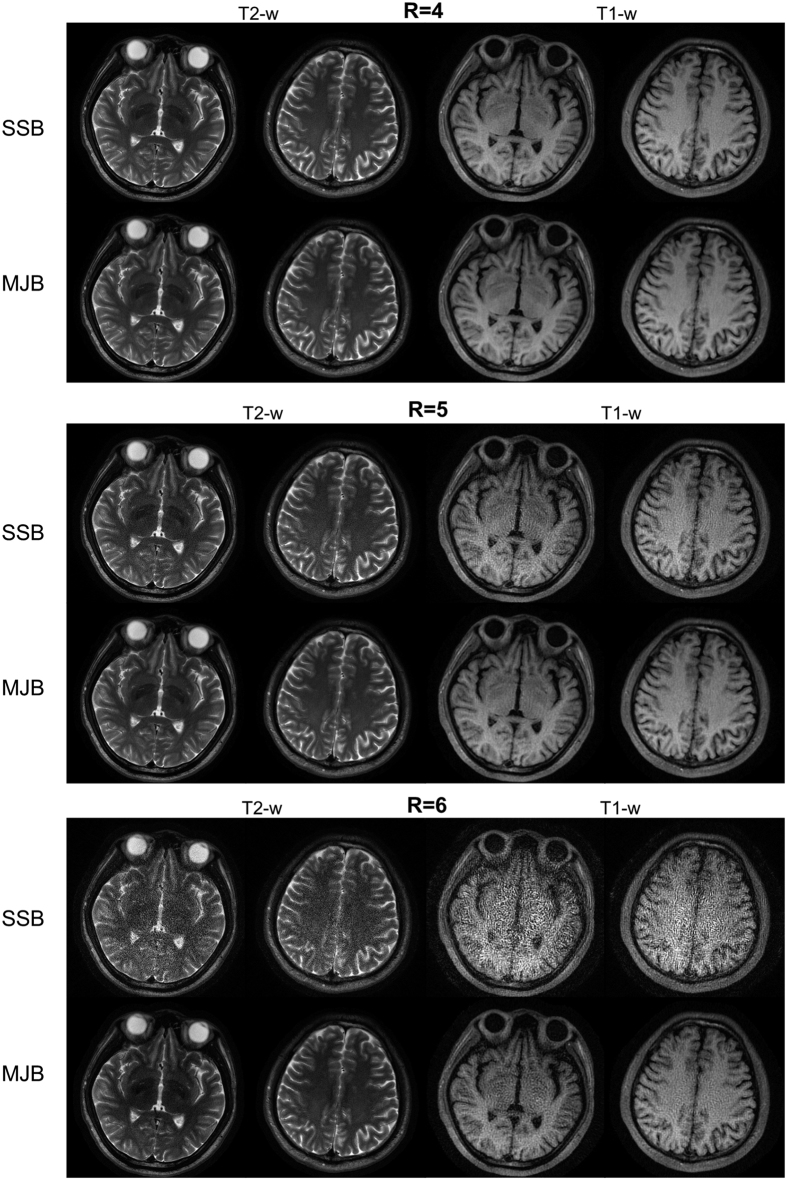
Images reconstructed from the *in vivo* brain data (without head motion) using PROPELLER with acceleration factor R = 4, 5 and 6 and an eight-channel coil. For both T1-weighted (T1-w) and T2-weighted (T2-w) imaging, MJB SENSE resulted in higher SNR than SSB SENSE. The improvement became increasingly apparent at higher acceleration factors. In particular, SSB SENSE failed to reconstruct meaningful images at acceleration factor of 6 whereas MJB SENSE still led to reasonably reconstructed images.
